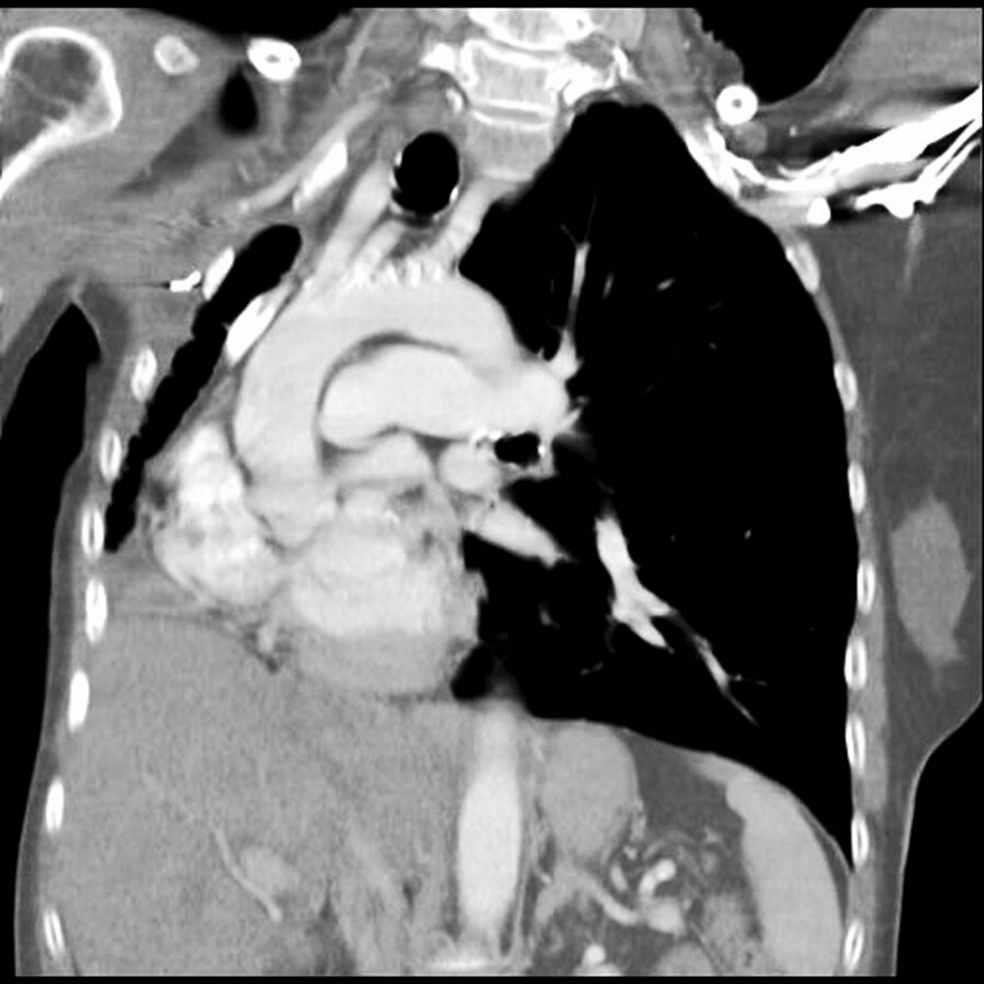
CT scan chest (pre-operative) Demonstrating marked volume loss of the right lung with bronchiectatic changes.
